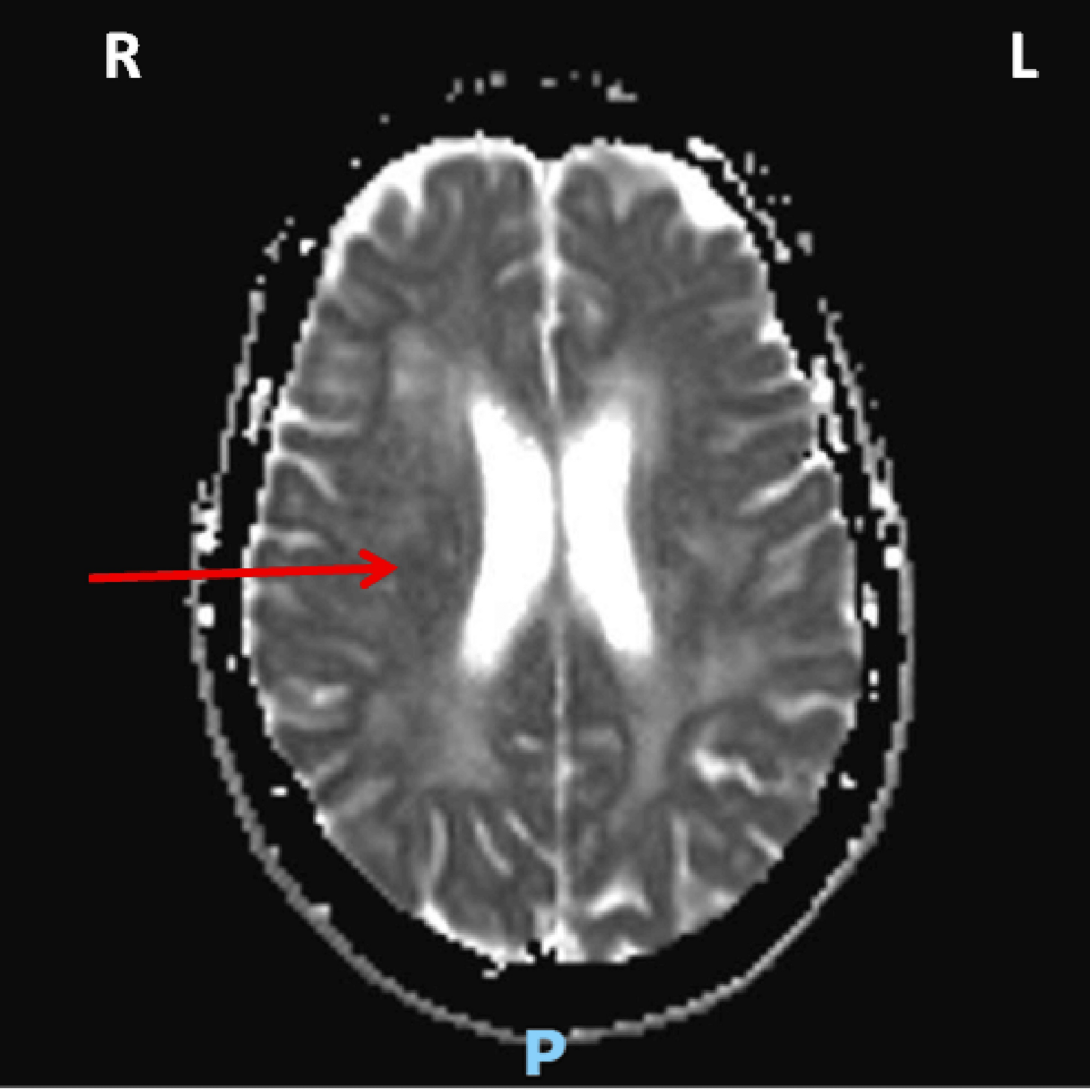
Corresponding lesion shown on axial MRI brain ADC confirming an acute right frontal lobe infarct (red arrow) MRI: magnetic resonance imaging; ADC: apparent diffusion coefficient
